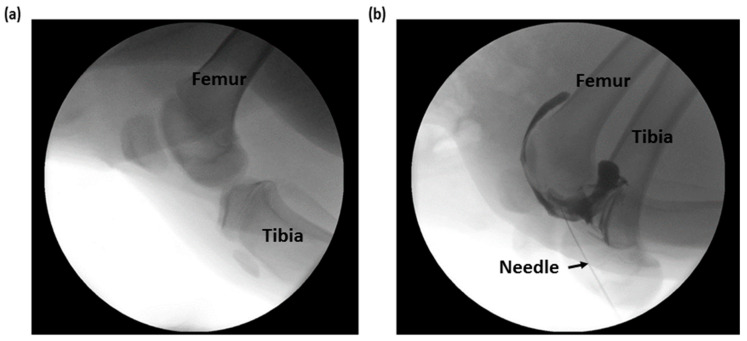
X-ray images by fluoroscopy (a) before and (b) after injection of contrast in the intra-articular space.
